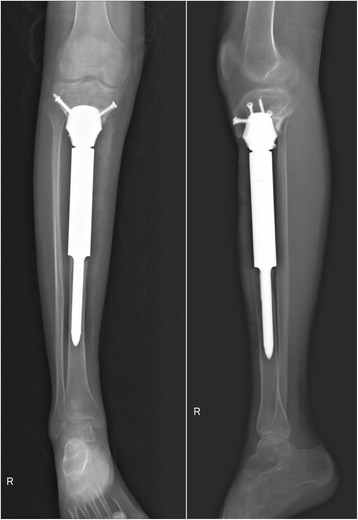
Anteroposterior and lateral radiographs after reconstruction with the 3D-printed prosthesis
